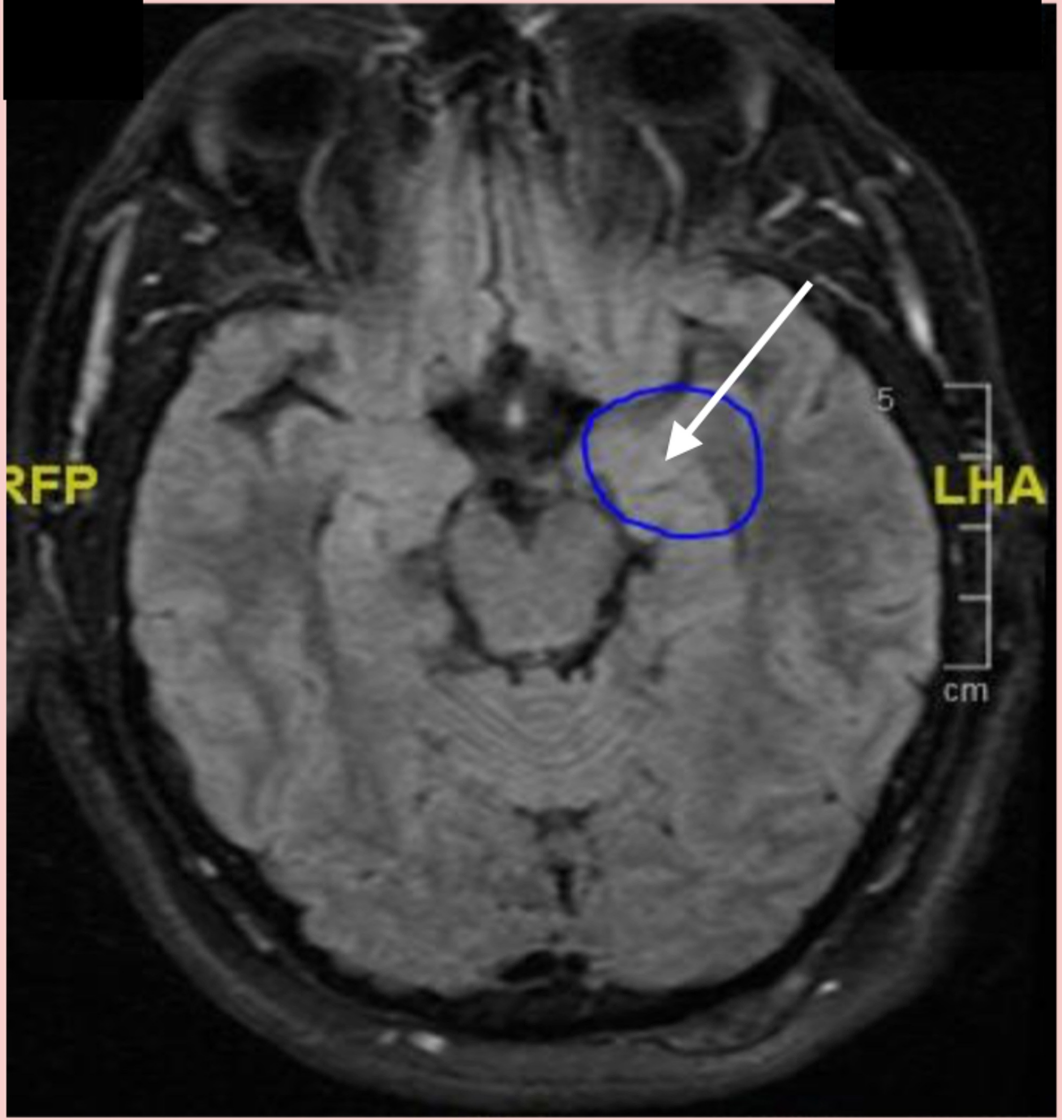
MRI brain showing amygdala enlargement.
